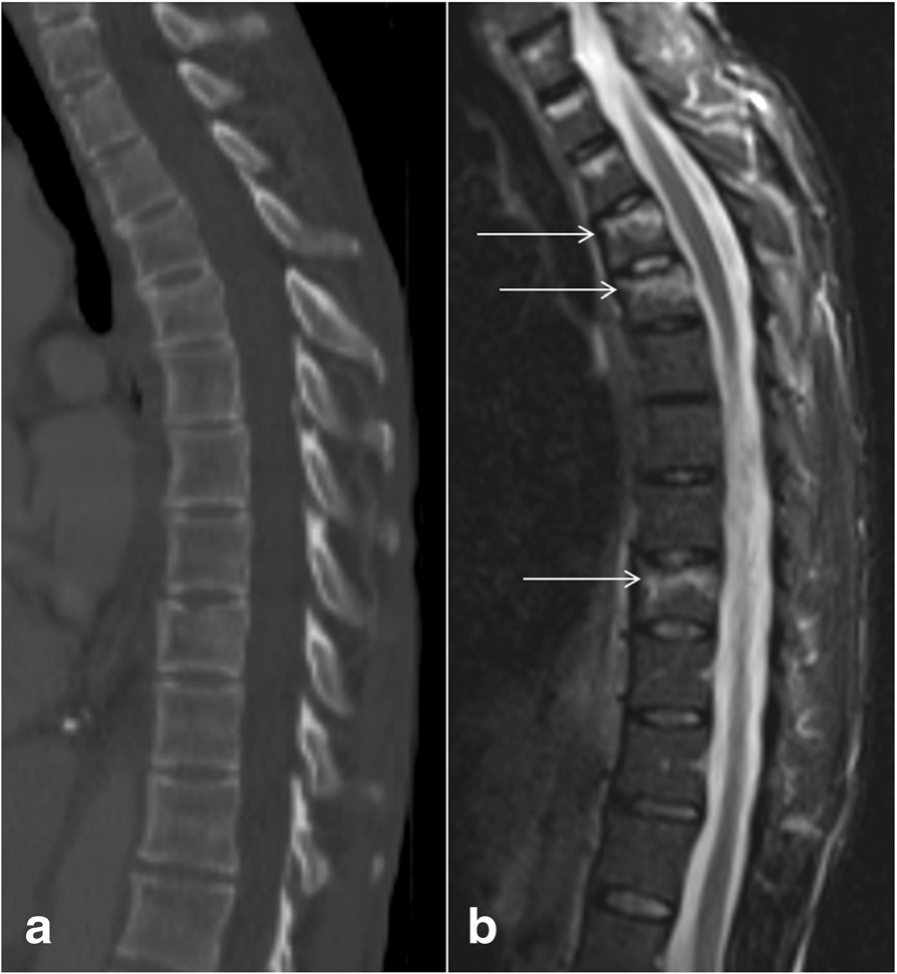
Sagittal CT image in the bone window (a) did not show any CT evidence for a fracture in this trauma patient. However, sagittal short tau inversion recovery (STIR) image (b) shows bone marrow edema in the superior aspect of multiple vertebrae (*arrows*) suggesting bone contusions
